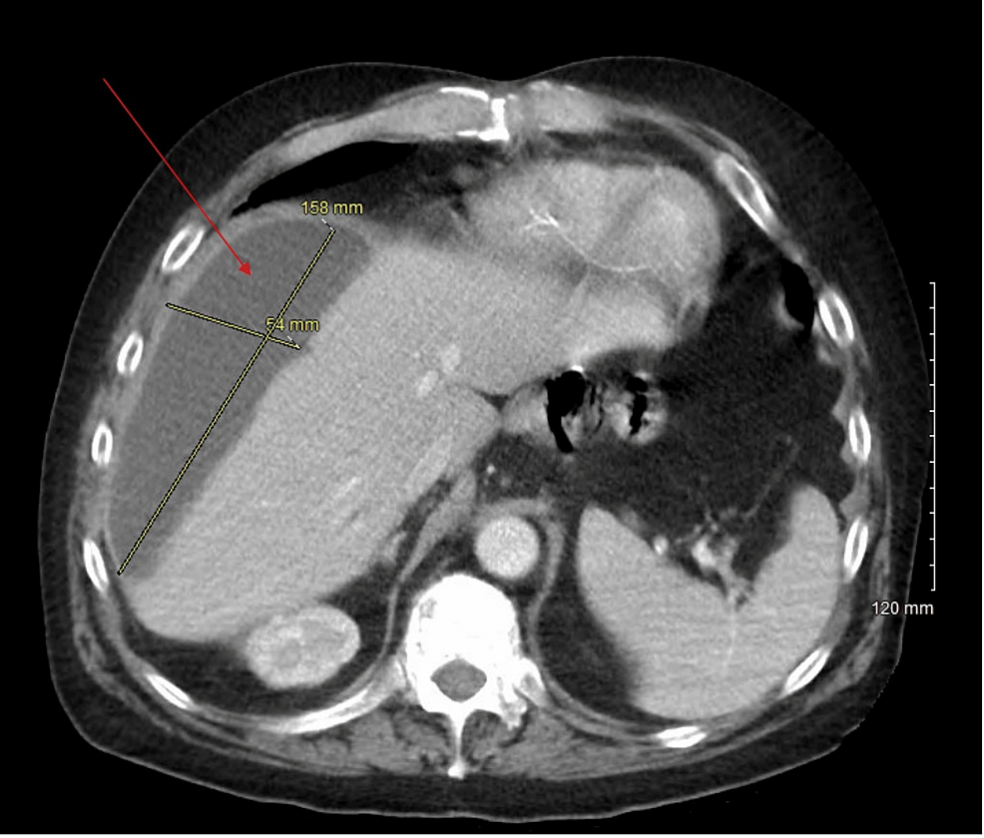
Axial CT scan of the abdomen showing subcapsular fluid collection in the right perihepatic space (red arrow). CT: computed tomography
